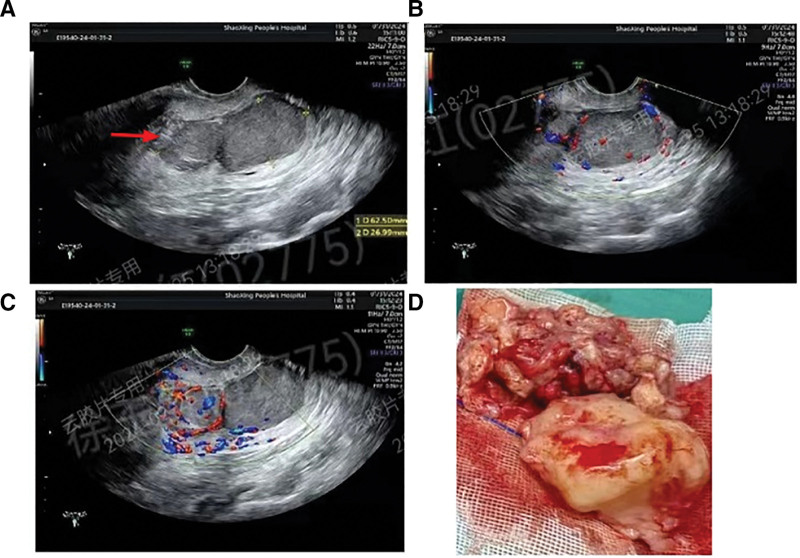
(A–C) Ultrasound images of the tumor. (D) The gross of the excised tumor.
